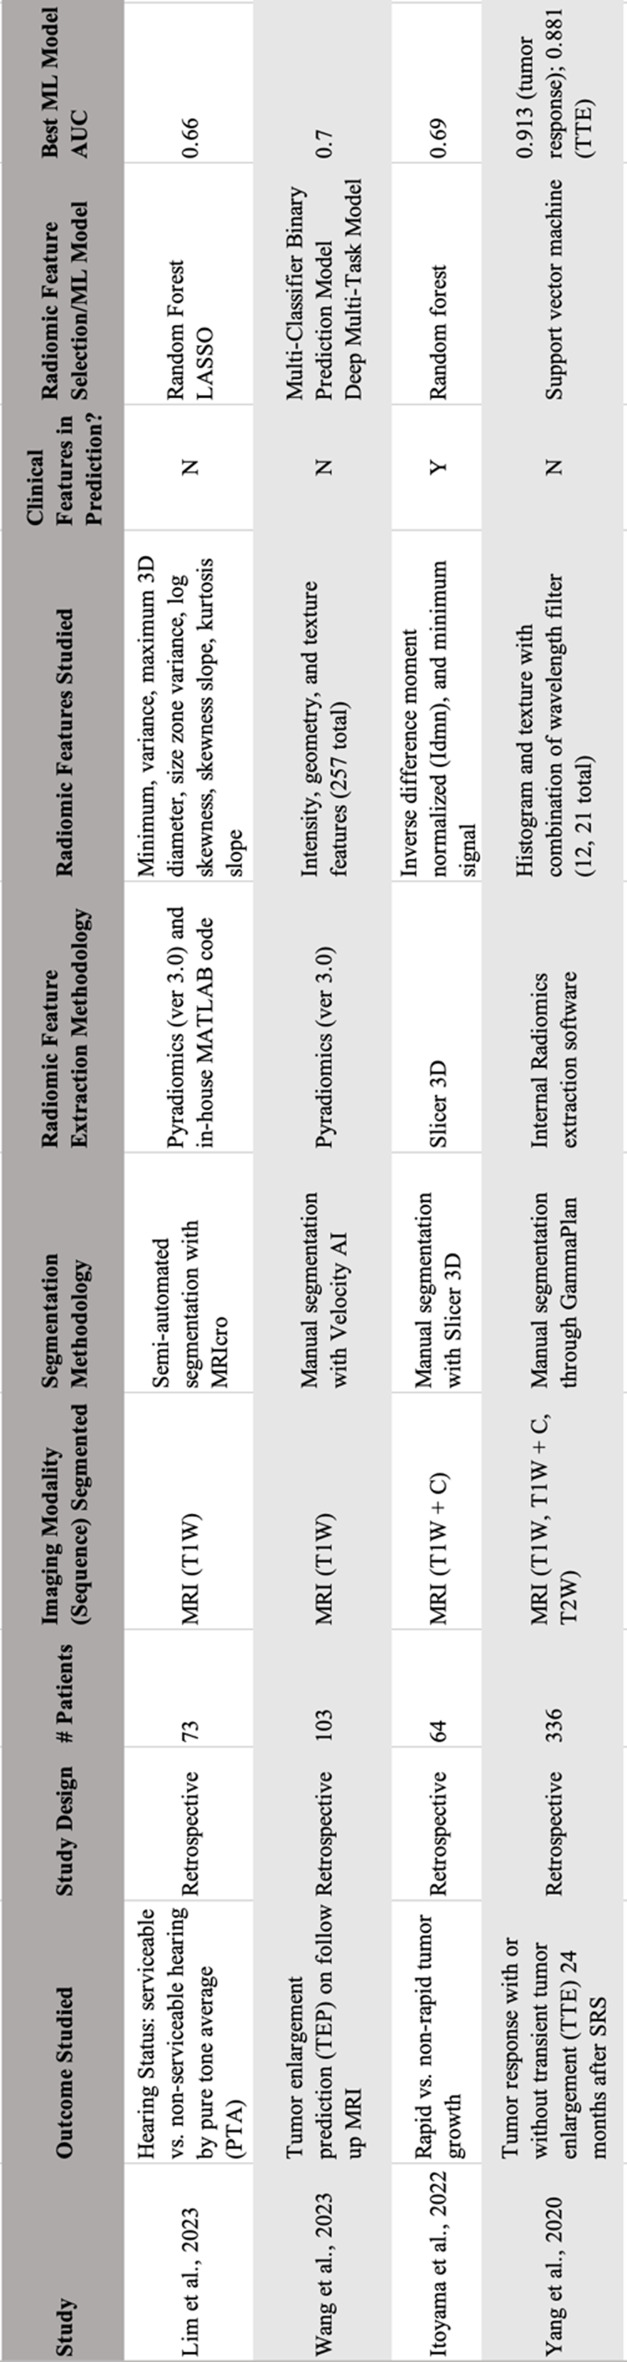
Overview of studies utilizing radiomic features in determination of pre-procedural clinical Outcomes in VS. Abbreviations: PTA = pure tone average; TEP = tumor enlargement prediction; LASSO = least absolute shrinkage and selection operator; MRI = magnetic resonance imaging; T1W = T1-weighted; T1W + C = T1-weighted with contrast
